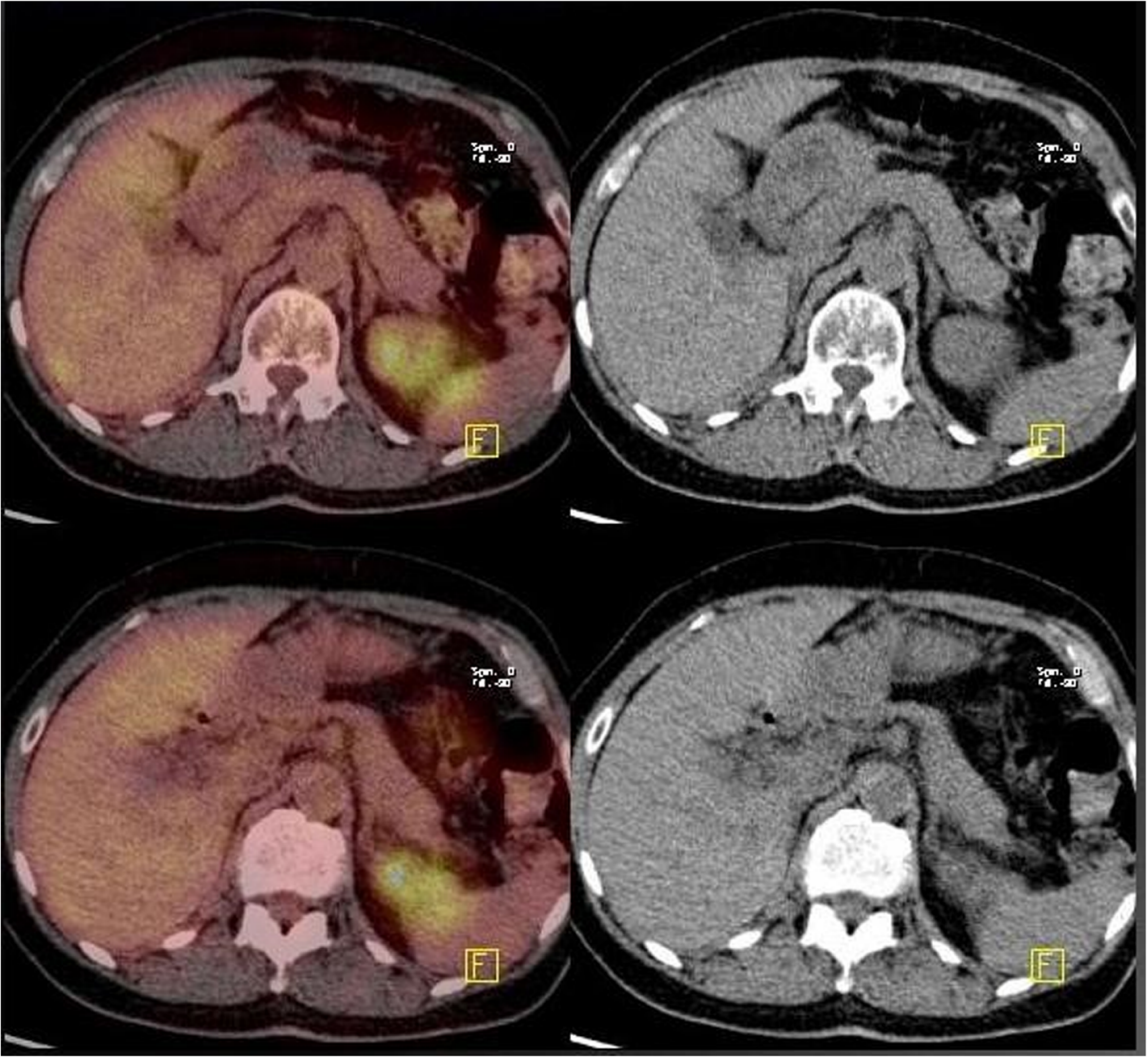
PET/CT incidental detection of left ureteral cancer associated with IPMN (adenoma) of the pancreatic tail (PET negative)
